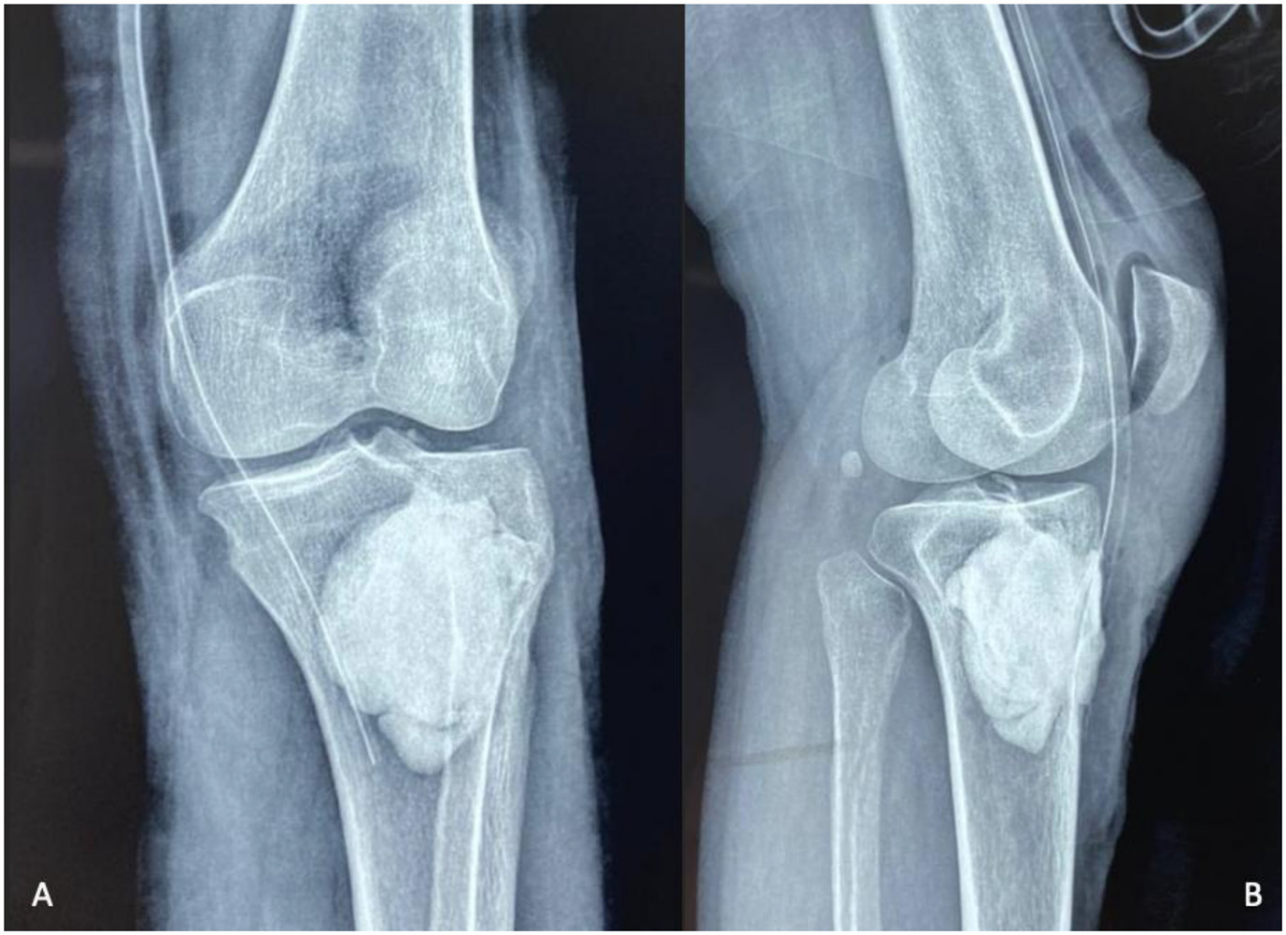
Postoperative control radiographs of the knee, anteroposterior (A) and lateral (B) views, showing the appearance after debridement and curettage of the lytic cavity, followed by filling with bone cement.
